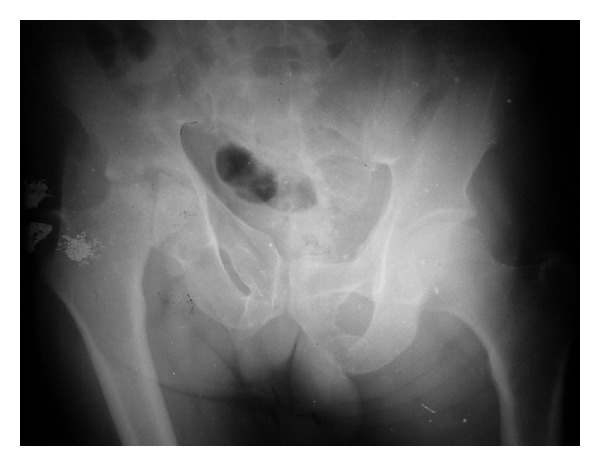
X-ray pelvis with both hips showing collapse of right femur head with fracture of head of femur. Evidence of old vertical shear type sacroiliac disruption right side is seen.
